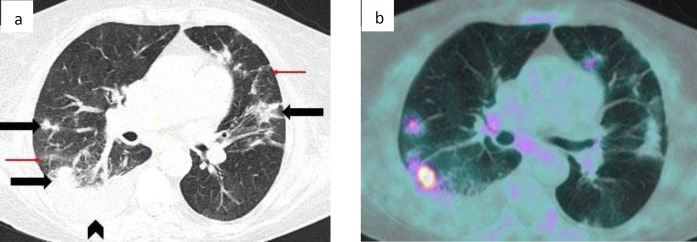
Female, 72 years old, with urothelial cancer. Axial CT image (a) shows bilateral compact consolidations (black arrows), mixed with peripheral GGO and reticulation (red arrows) and right pleural effusion (black arrowhead); findings consistent with a peak stage. On corresponding PET scan (b) SUV_max_ of the nodular consolidation adjacent to the pleural effusion was 7.7. The patient was a poorly compliant patient, but the family members did not report any symptom in the days before the scan
